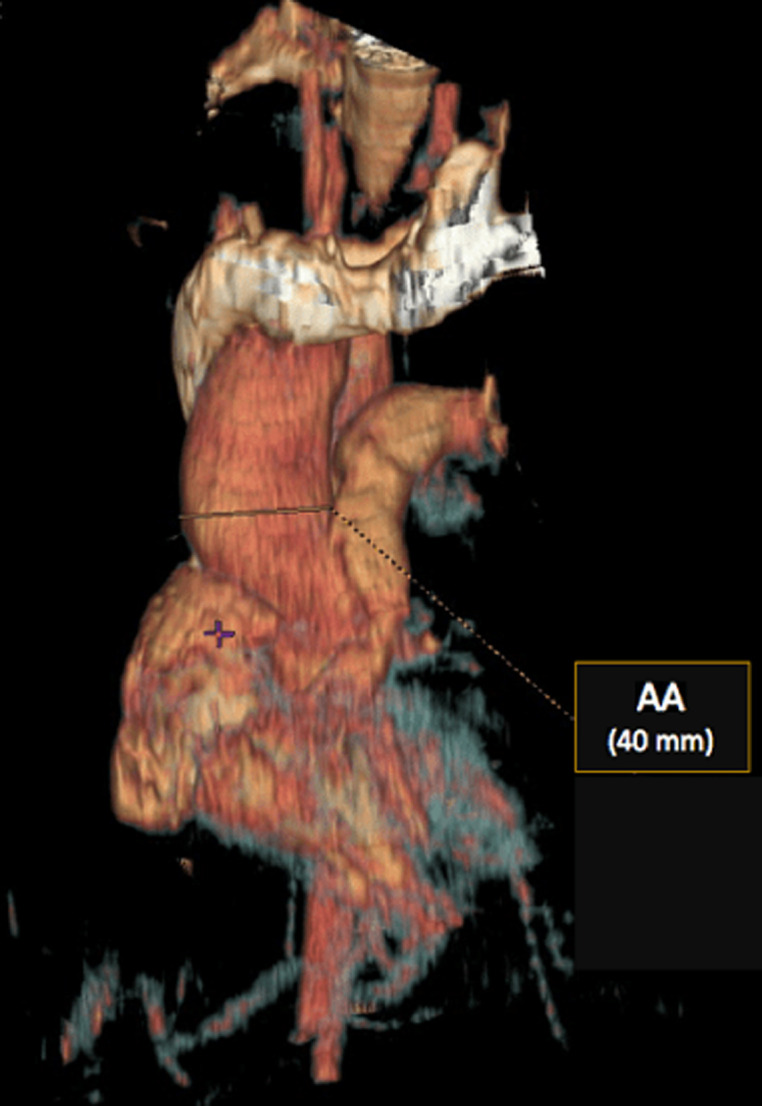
3D contrast-enhanced CT image demonstrating ascending aorta dilation with right aortic arch
